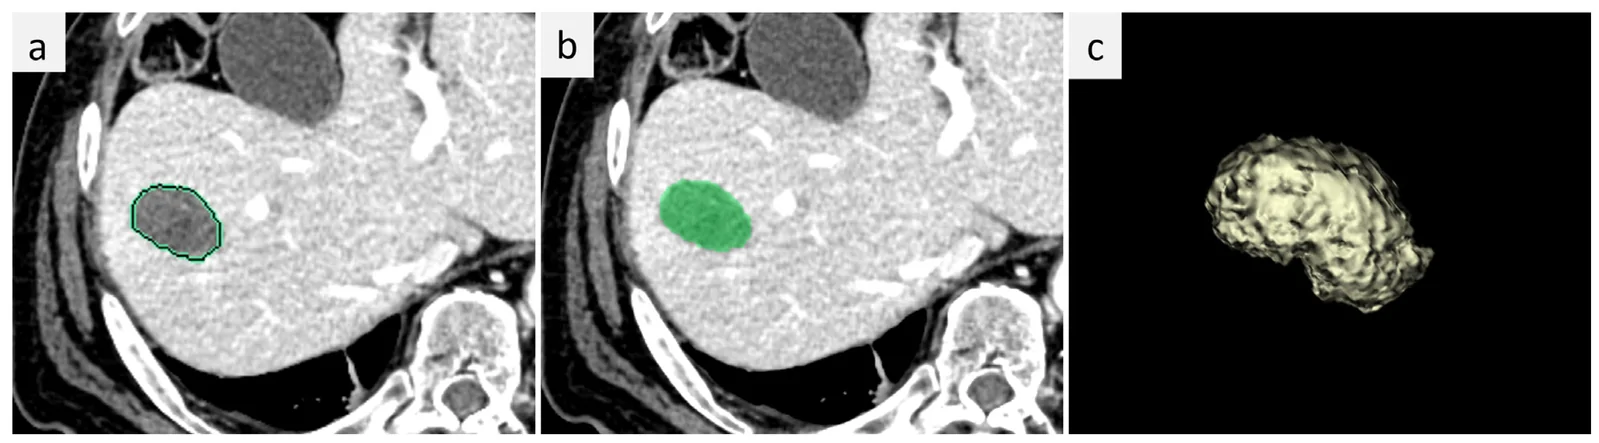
3D volumetric CT measurements of the ablated area in the liver ((a) manually traced image, (b) automatically summed image, (c) 3D image). The green contour in (a) indicates the manually traced boundary, and the green shaded area in (b) represents the corresponding segmented volume.
